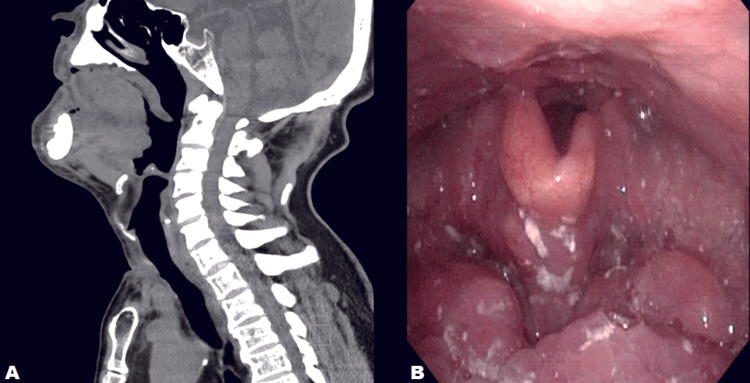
Postoperative airway evaluation A. Postoperative sagittal CT MPR showing complete resolution of oropharyngeal airway obstruction; B. Fiberoptic laryngoscopy after surgery demonstrating a clear, unobstructed laryngeal inlet with restored epiglottic mobility. MPR: multiplanar reconstruction
